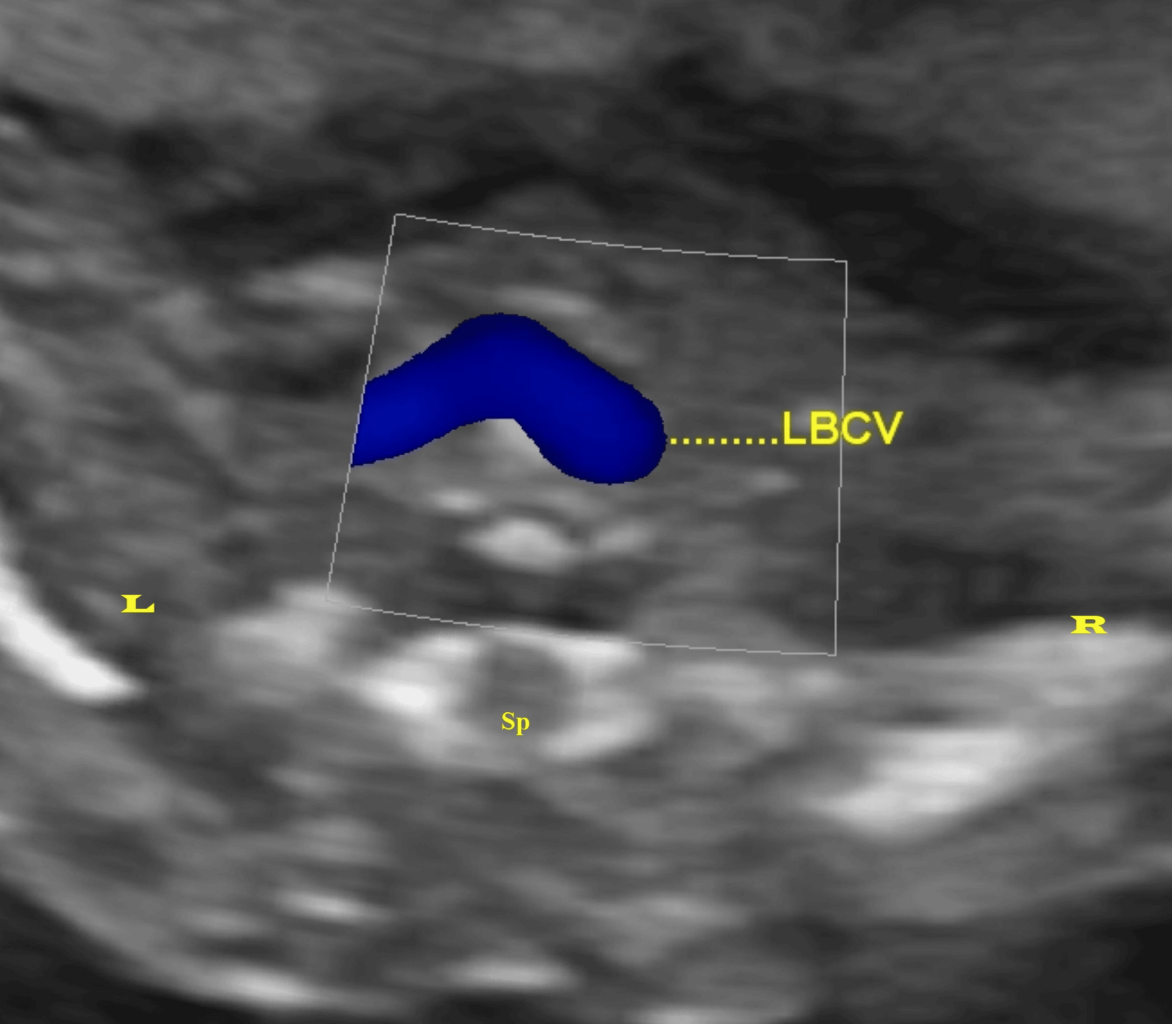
Color doppler ultrasound imaging of the FLBCV LBCV: left brachiocephalic vein, FLBCV: fetal left brachiocephalic vein, Sp: spine, L: left, R: right
